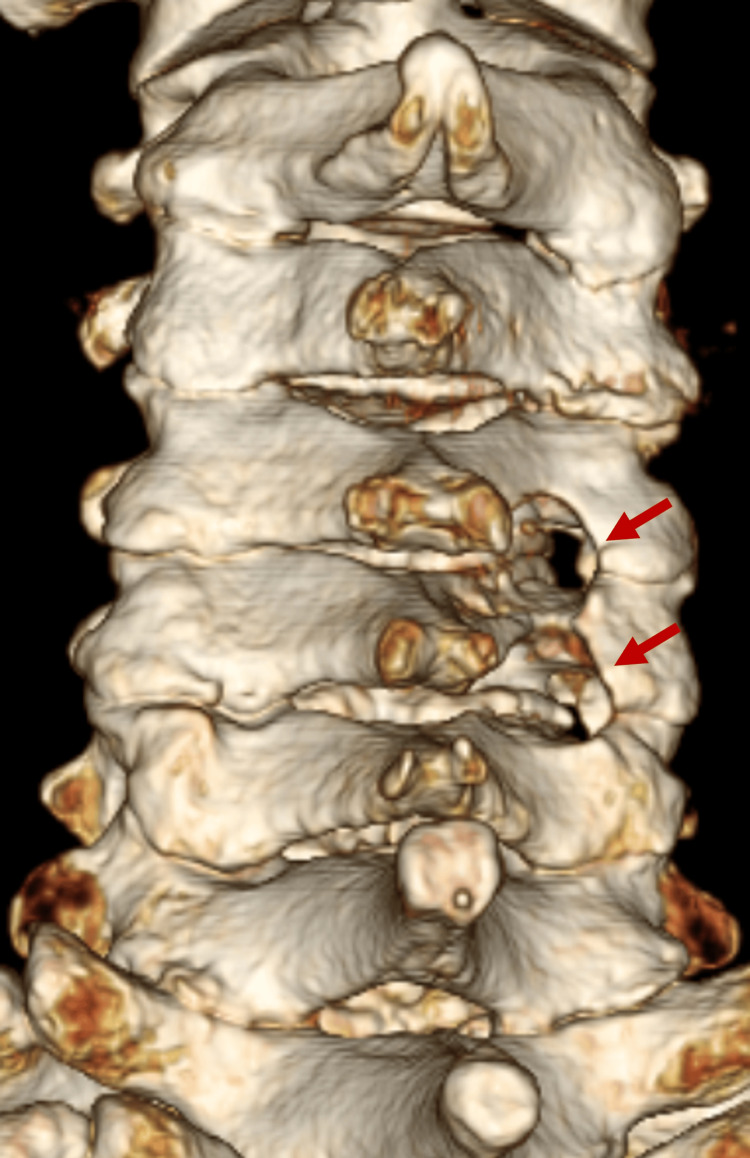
Postoperative 3D-CT image Posterior decompression is achieved beyond the Luschka joint. The arrows show the decompression sites CT: computed tomography
